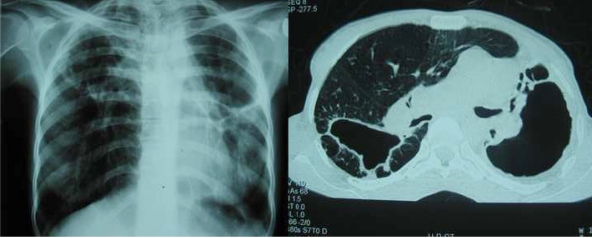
Chest Radiography, PA, and Conventional CT Scan on July 2007, Demonstrate bilateral fibrocavitary destructive changes and biapical pleural thickening.
